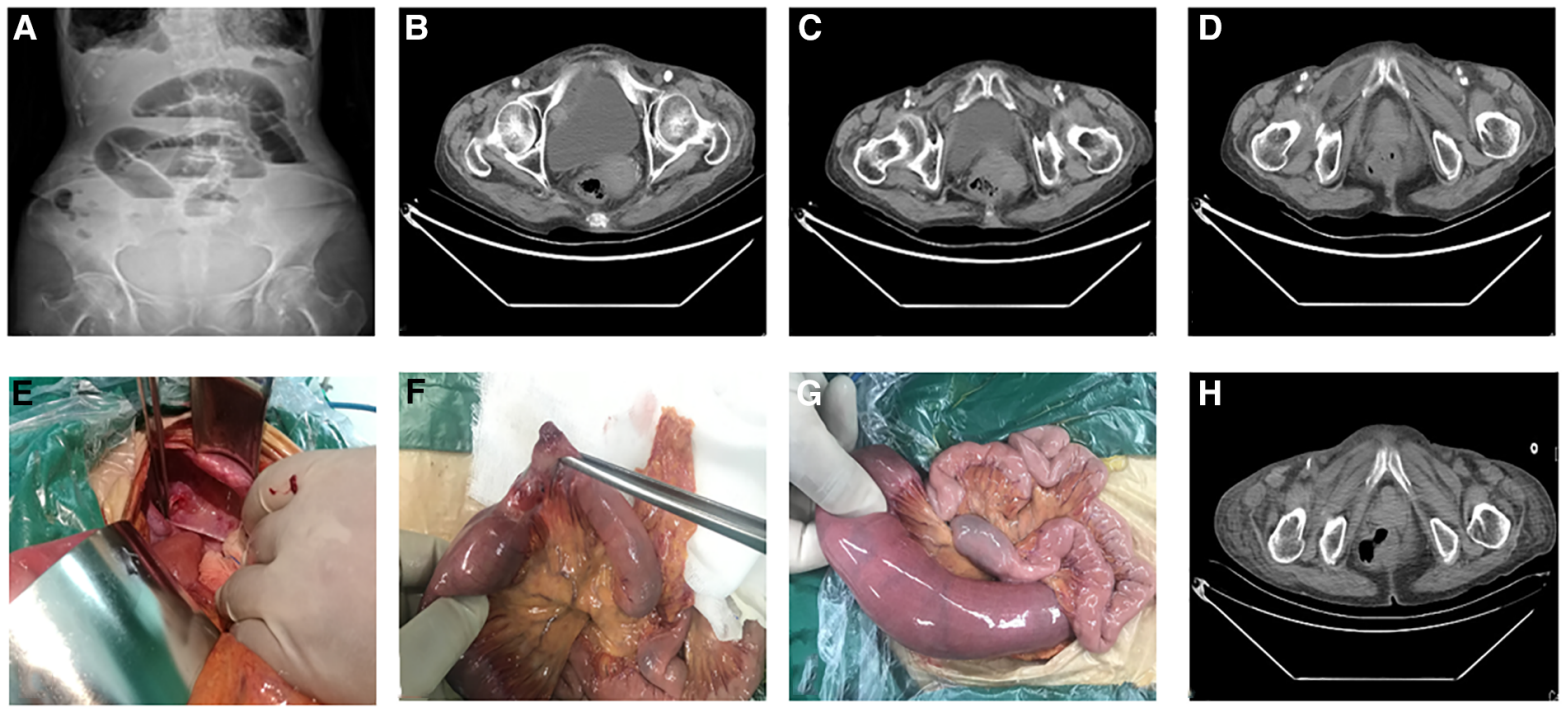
(A) Preoperative vertical radiographs of the abdomen showing the small bowel obstruction; (B–D) preoperative pelvic CT showing intestinal hernia in the obturator foramen; (E–G) Ischemic necrosis occurred in part of the small intestine and herniated into the obturator foramen during the operation; (H) Pelvic CT reexamination showed that the obturator foramen had returned to normal.
